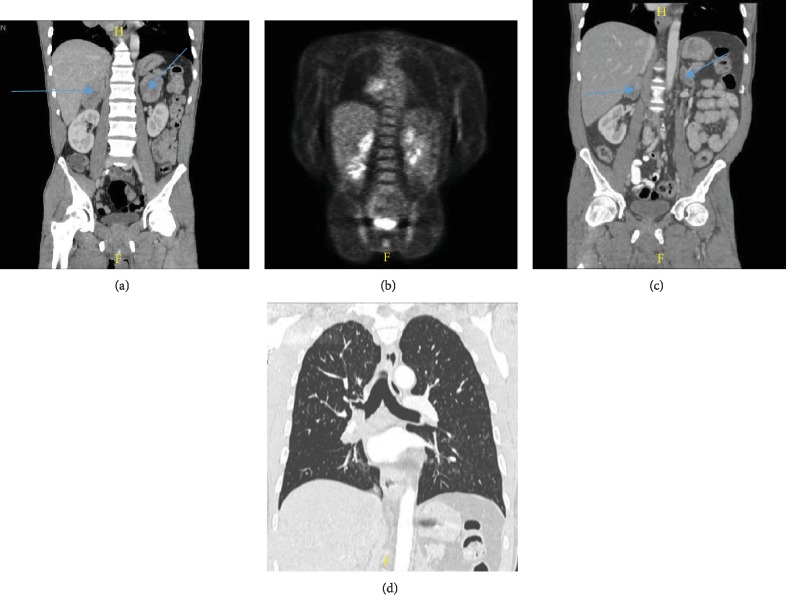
(a) CT abdomen and pelvis with IV contrast showing bilateral adrenal masses with the right measuring 5.4 × 4.0 cm and the left measuring 4.8 × 2.0 cm, highly concerning for metastatic disease. Arrows pointing to adrenal masses. (b) PET CT prior to chemotherapy showing metabolically active right hilar mass, metabolically active right paratracheal lymphadenopathy, decreased prominence of mediastinal adenopathy, and metabolically active bilateral adrenal masses consistent with metastatic disease. (c) CT abdomen and pelvis with PO and IV contrast demonstrates that both adrenal glands have significantly decreased in size, measuring 2.9 × 1.6 cm on the left and 3.4 × 2.0 cm on the right. Arrows pointing to adrenal masses. (d) CT of the chest revealing near-total resolution of the right hilar mass without postobstruction.
